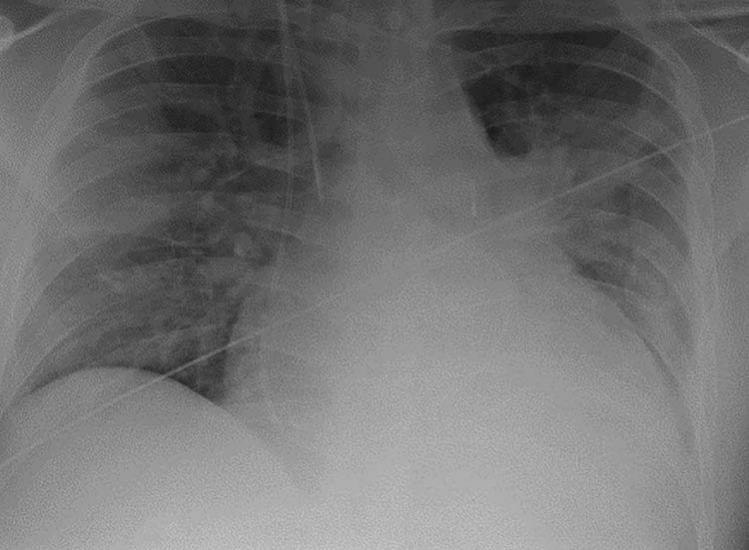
Chest radiograph demonstrating pulmonary oedema prior to implantation of biventricular assist device.
